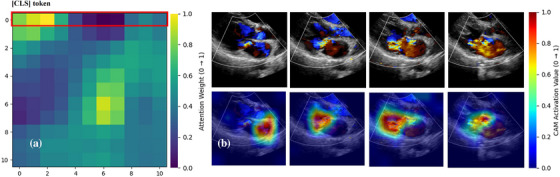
Activation maps of the attention‐based MR Detection model in Case 2 (PLAX‐Color). (a) Self‐attention matrix covering 11 input frames, where index 0 corresponds to the [CLS] token. MR events occur between frames 1–5; row 0, highlighted by the red box, shows the attention weight assigned to each frame by the [CLS] token—warmer colors indicate higher attention (see color bar on the right). The model shows high attention to frames 1–4, which happen to be MR frames. (b) Top row: Raw color Doppler echocardiographic frames from the MR sequence. Bottom row: Corresponding attention heatmaps superimposed on the same frames. The heatmaps show that the model can pay attention to the color Doppler information corresponding to the position of the mitral valve.
